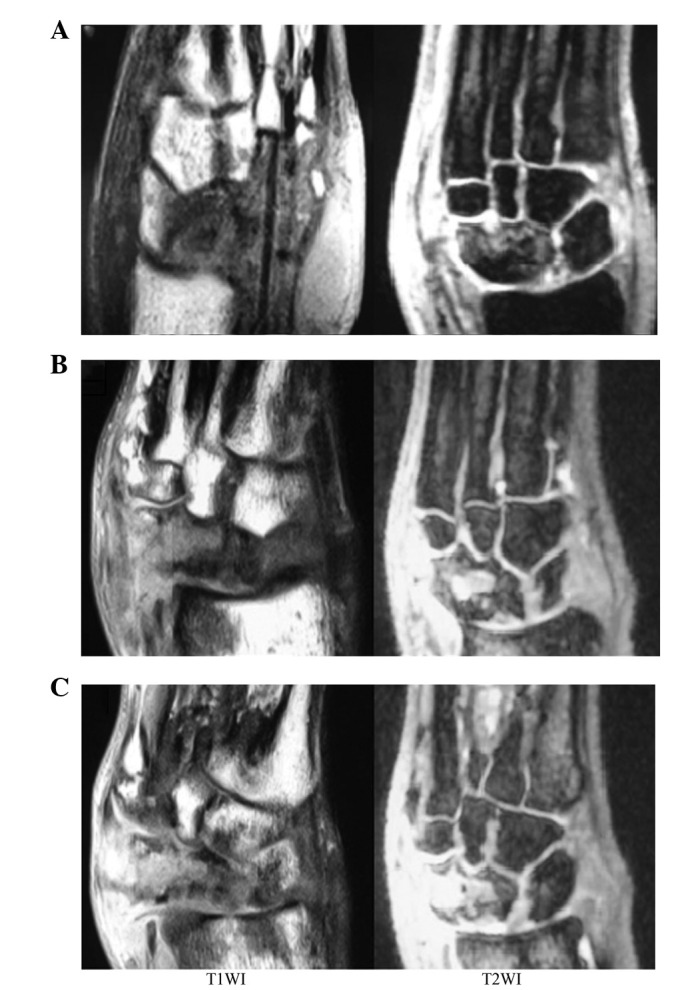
Images by magnetic resonance imaging (MRI) detection. (A) Images in week 3 after surgery. Hypointense foci were observed on T_1_-weighted images (T_1_WI) and disseminated punctate hyperintense foci were observed on T_2_WI. (B) Images in week 6 after surgery. Changes in the hypointense images on T_1_WI and hyperintense images on T_2_WI were observed. (C) Images in week 12 after surgery. A large area of hypointensity was detected on T_1_WI and a large area of hyperintensity was detected in the lunate on T_2_WI, within which disseminated areas of punctate hypointensity (necrotic bone chips) were embedded.
